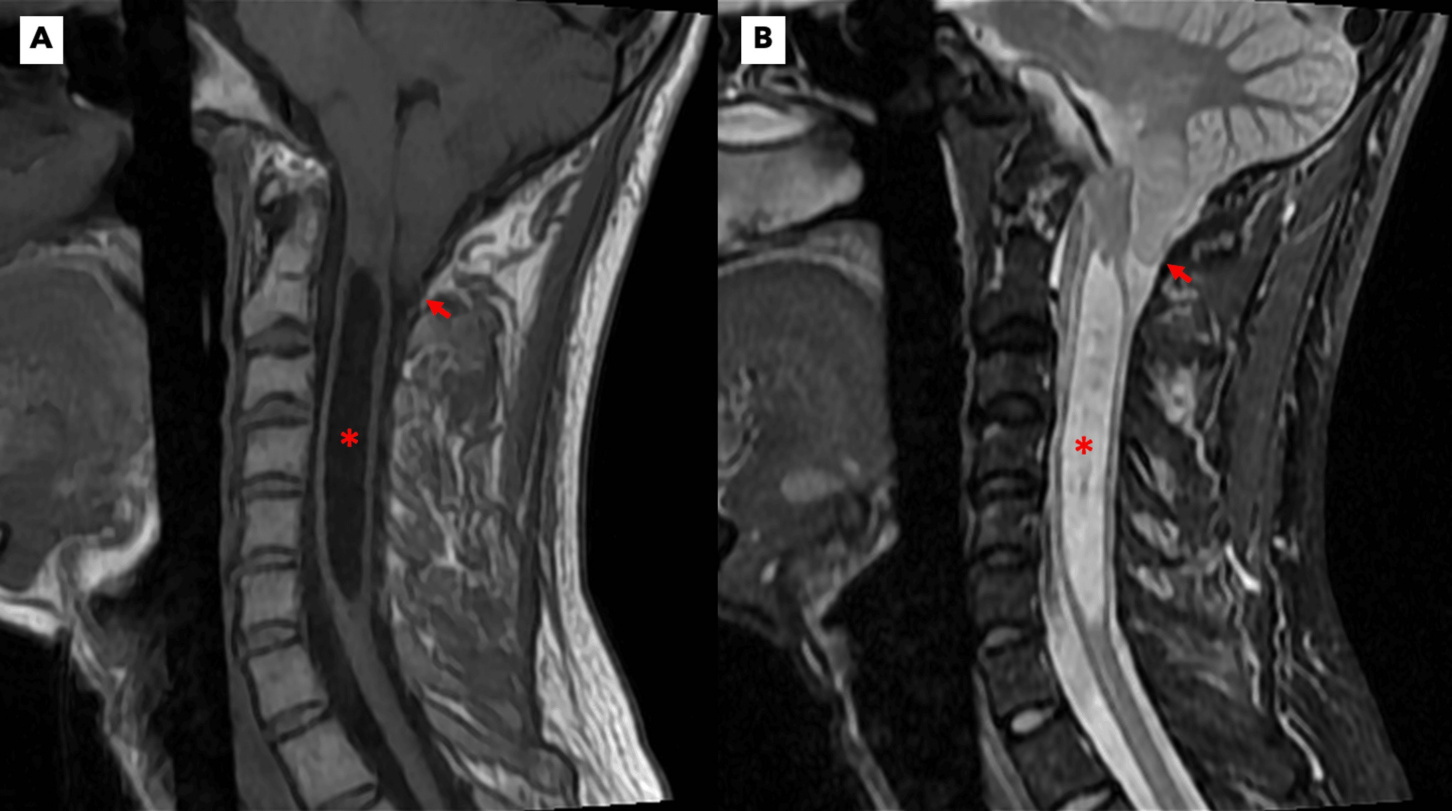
Pre-operative cervical MRI findings Sagittal T1 (A) and T2 (B) weighted MRI demonstrate herniation of the cerebellar tonsils through the foramen magnum (arrows). A large, centrally located syrinx (stars) is seen spanning C1 to C6, causing expansion of the spinal cord. These findings are consistent with ACM1. ACM1: Arnold-Chiari malformation type I; MRI: magnetic resonance imaging
